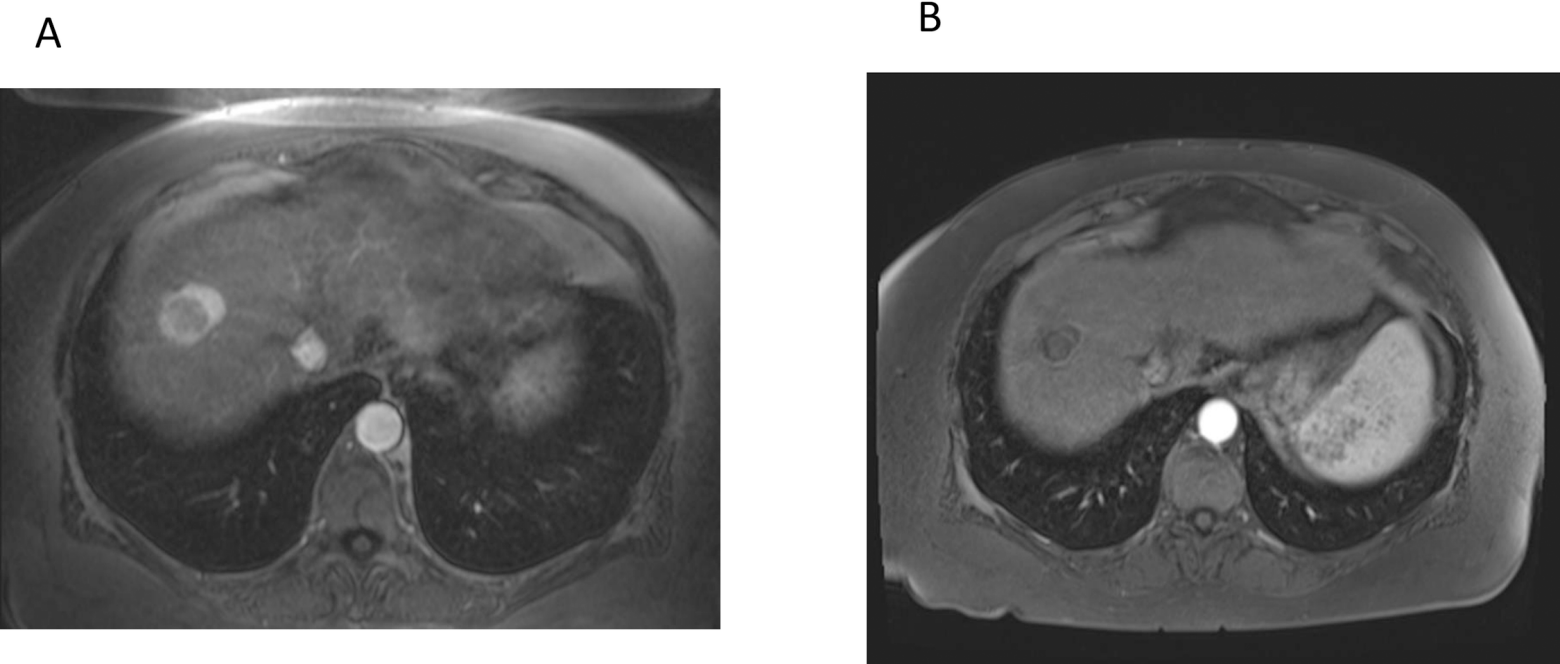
(A) Initial MRI demonstrating a 3.5 cm hyperenhancing right lobe mass, LIRADS-5 for HCC. (B) Post embolization MRI demonstrating complete response to treatment.
